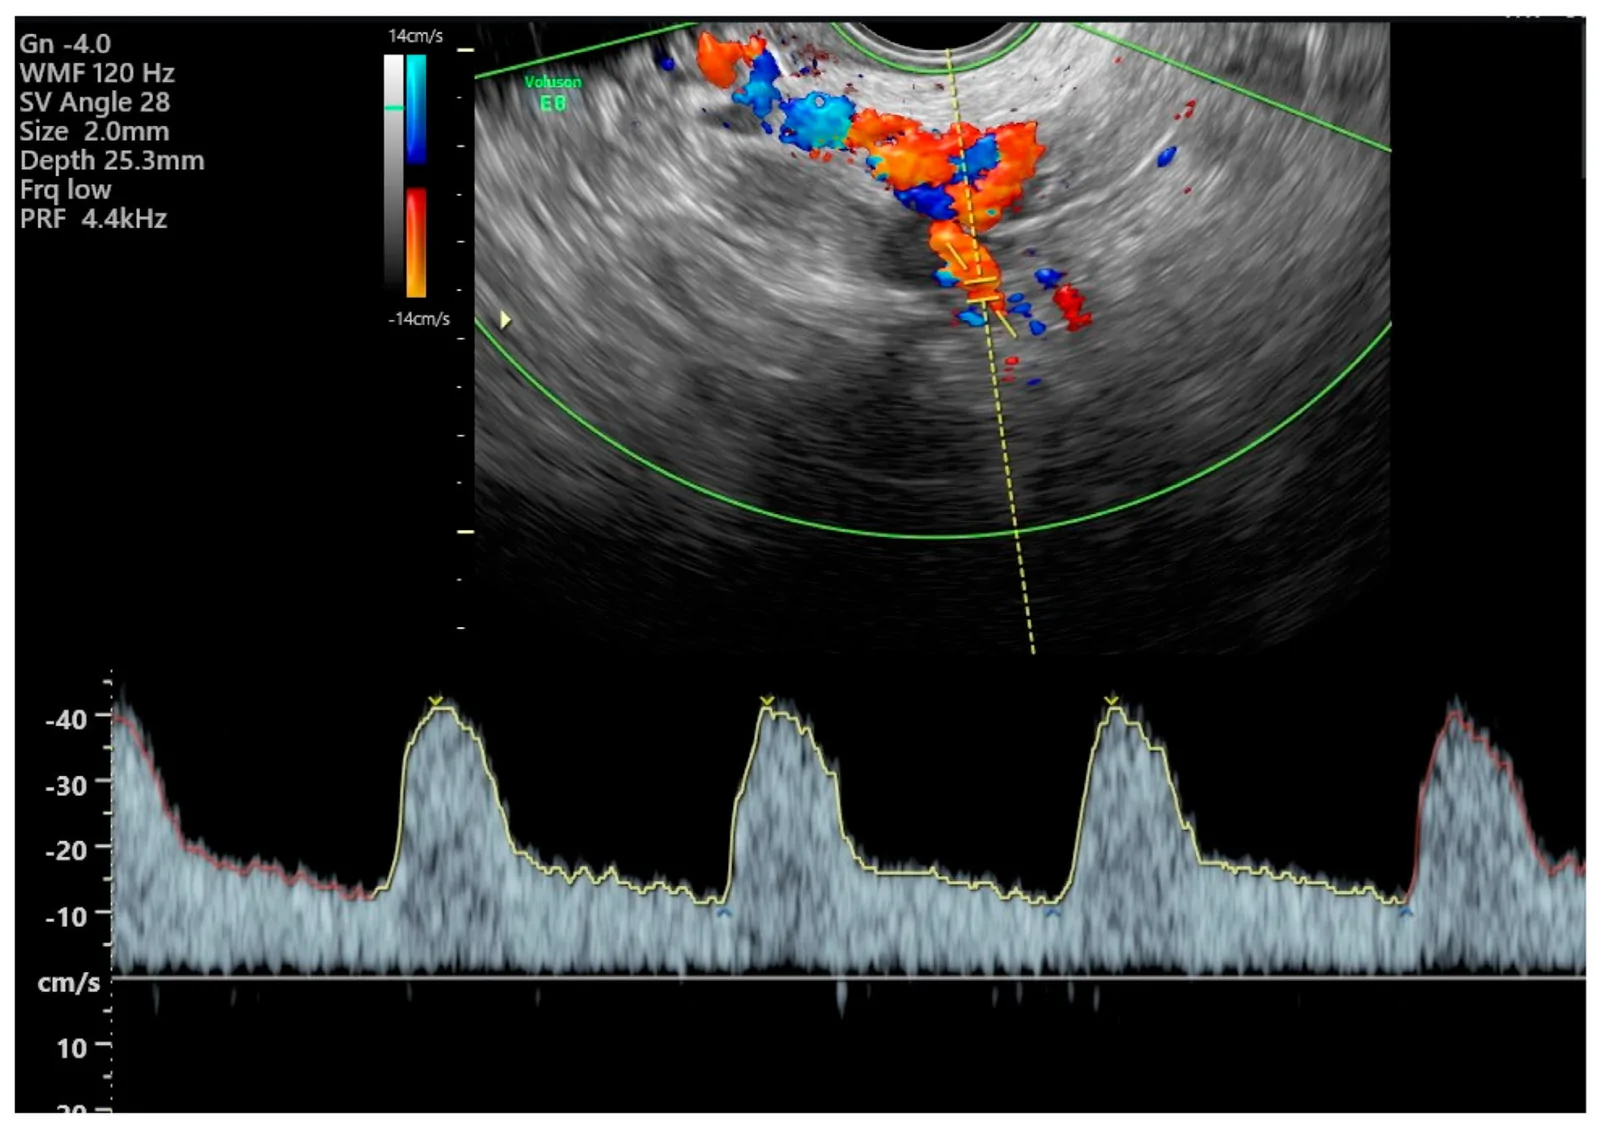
The Power Doppler examination of the uterine artery in a 47 year old patient diagnosed with FIGO stage IB2 mucinous adenocarcinoma of cervix.
